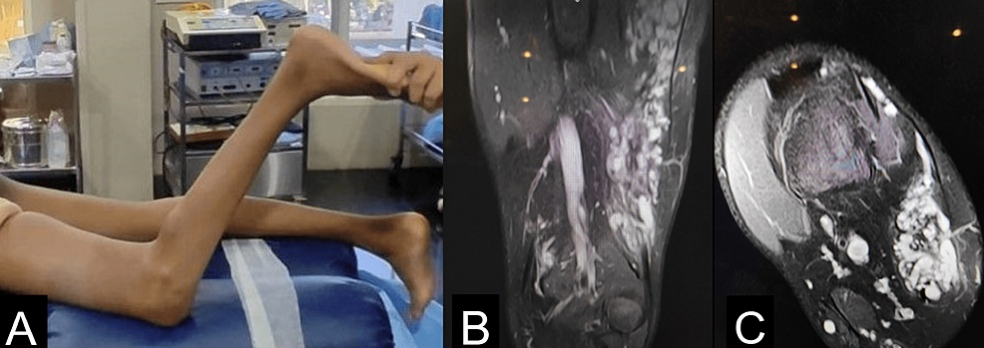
Intramuscular venous malformation (IMVM) involving the left hamstrings in our patient demonstrating (A) pre-operative severe fixed flexion deformity, Magnetic resonance images of involved leg showing (B) multiple engorged tortuous venous structures involving the quadriceps muscles with abnormal venous contrast enhancement (C)
